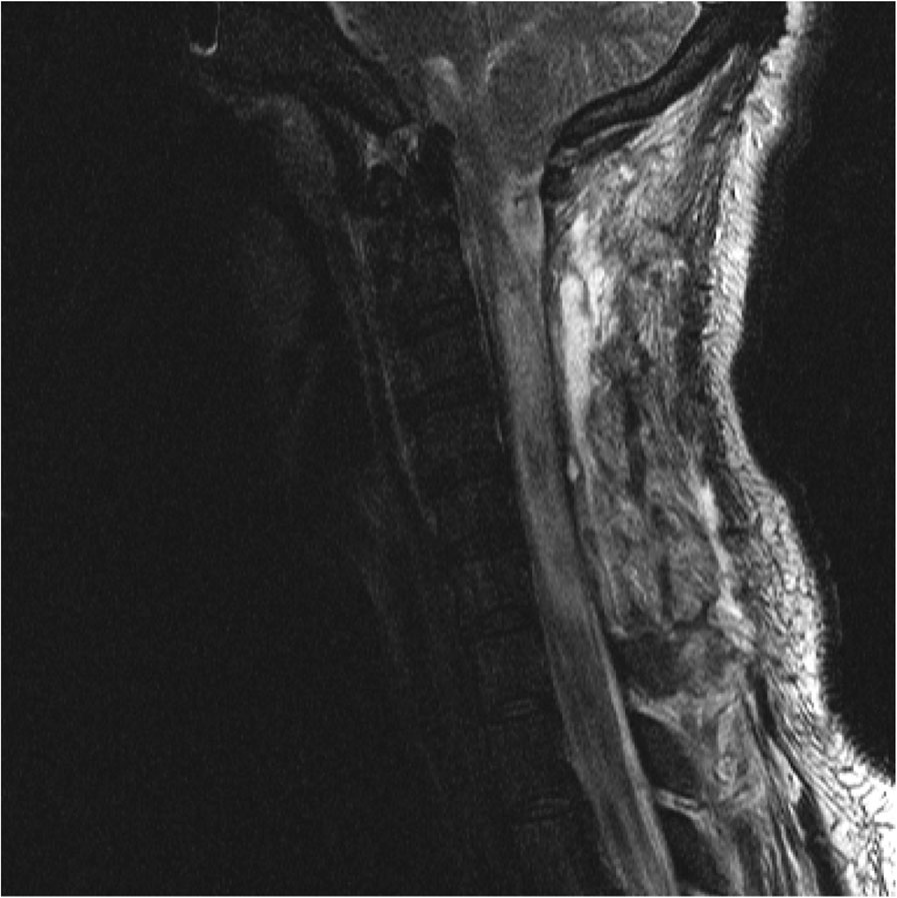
Magnetic resonance imaging scan of the cervical spinal cord of the intramedullary abscess obtained 9 days postoperatively. This sagittal T2-weighted image shows stable postoperative changes after a myelotomy and laminectomy, as well as a persistent but stable T2-weighted hyperintensity starting from the level of C2 and extending down to the level of T1
